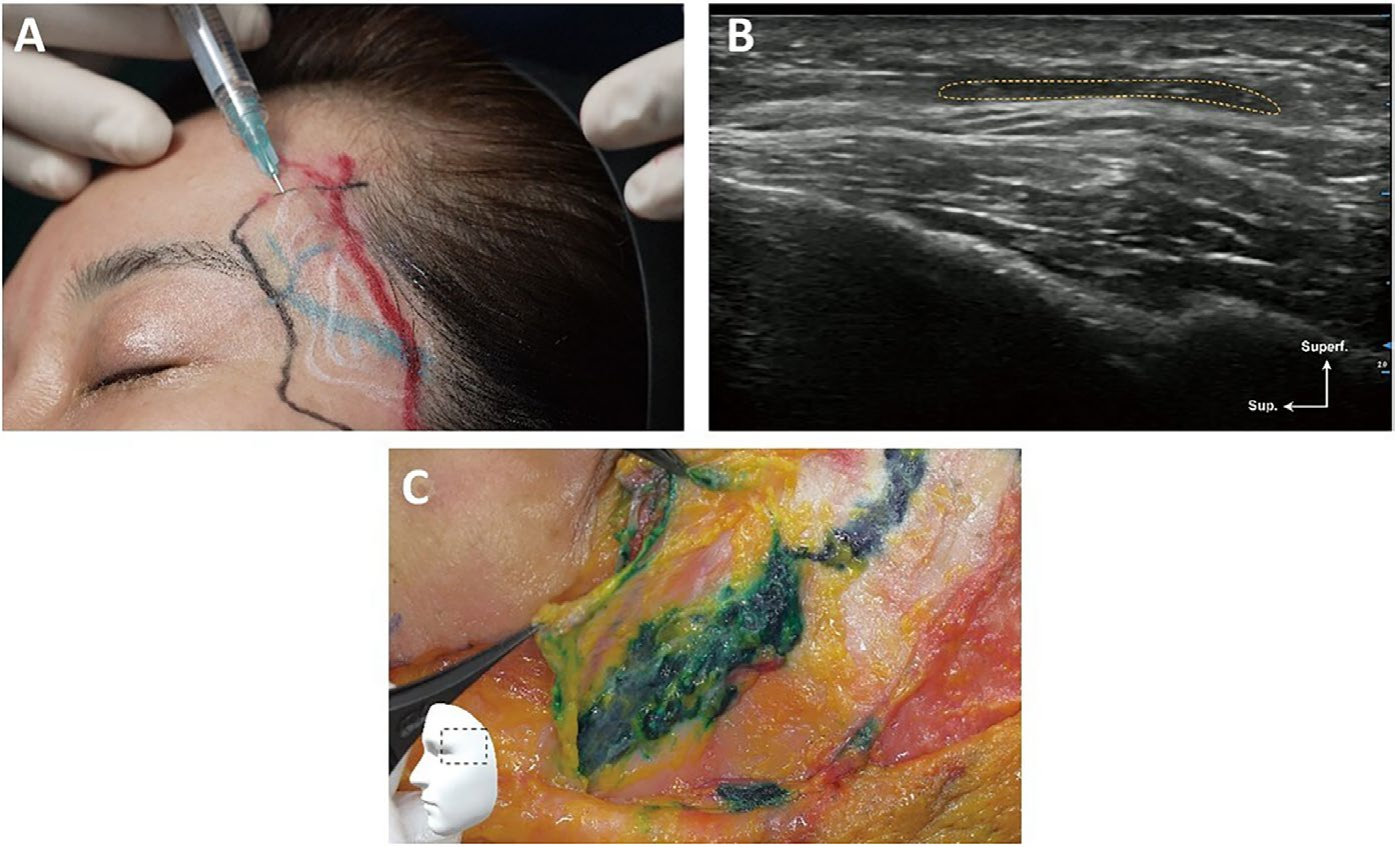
A demonstration of the superficial plane injection technique in the temple (41‐year‐old Korean female). (A) Clinical injection. (B) Clinical ultrasound. (C) Cadaver dissection (72‐year‐old Korean male).
